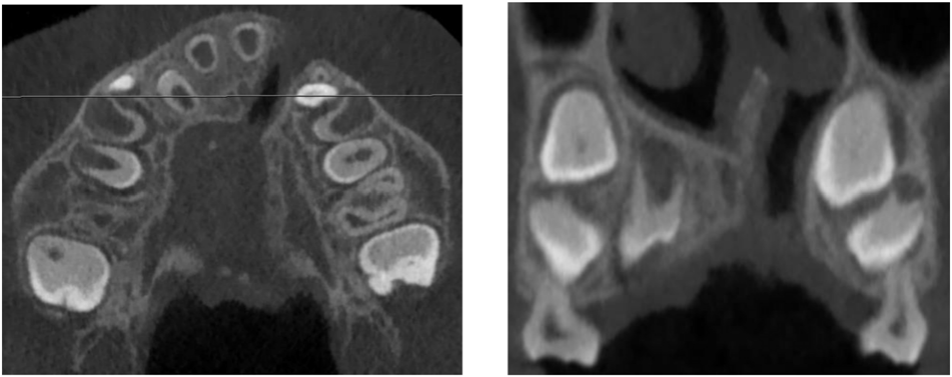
Low‐resolution CBCT of the maxilla with FOV 8 × 5 cm^2^ in an 8‐year‐old patient with unilateral cleft lip and palate for preoperative SABG planning. Axial slice (left) with the corresponding coronal slice (right) indicated by the line on the axial slice
